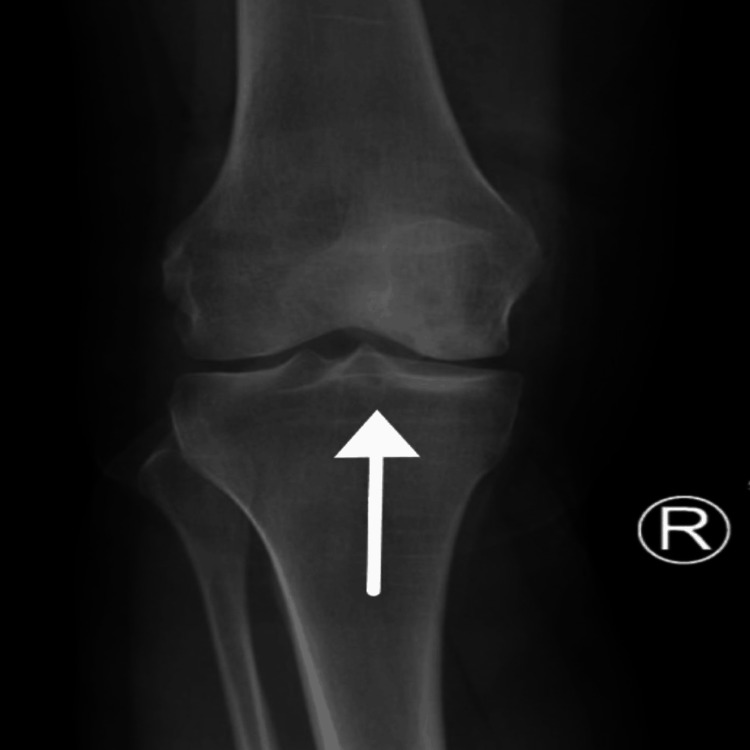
Pre-operatve X-ray of the right knee A white arrow highlights a radiolucent area on the anteroposterior aspect of the medial femoral condyle
